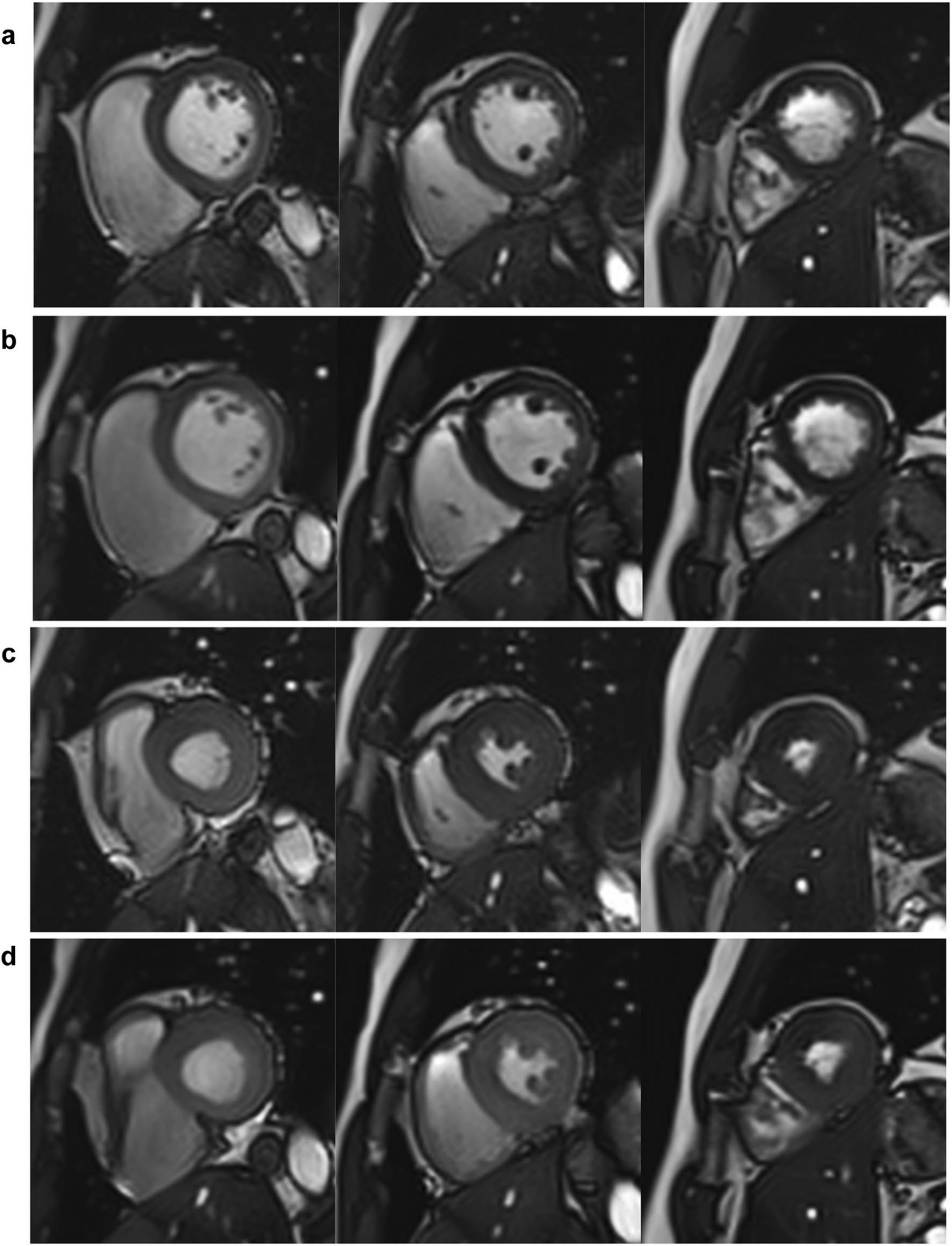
Comparison of representative cine MR images in end-diastole (a BH conventional cine images, b FBCS cine MoCo images) and end-systole (c BH conventional cine images, d FBCS cine MoCo images). Both observers rated the image quality as excellent (i.e., score 5) in both images
